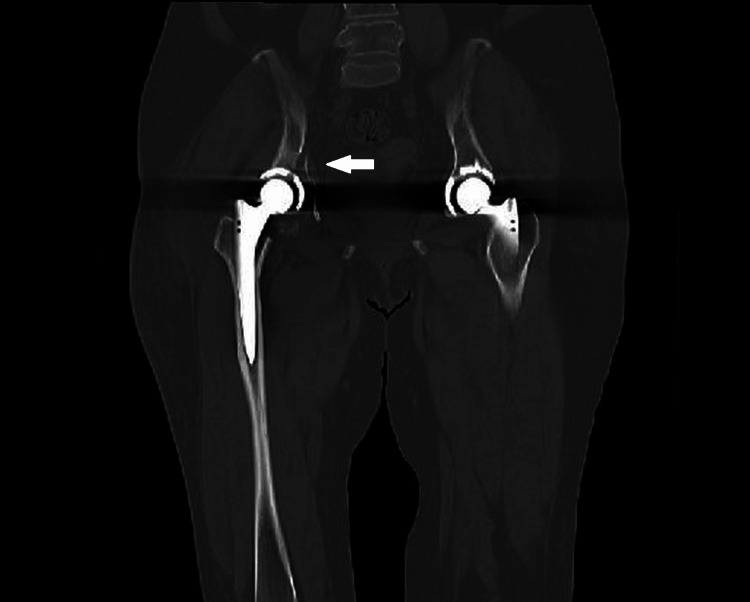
Pelvic coronal CT image at initial presentation. No clear evidence of loosening of the cup or stem was observed.
